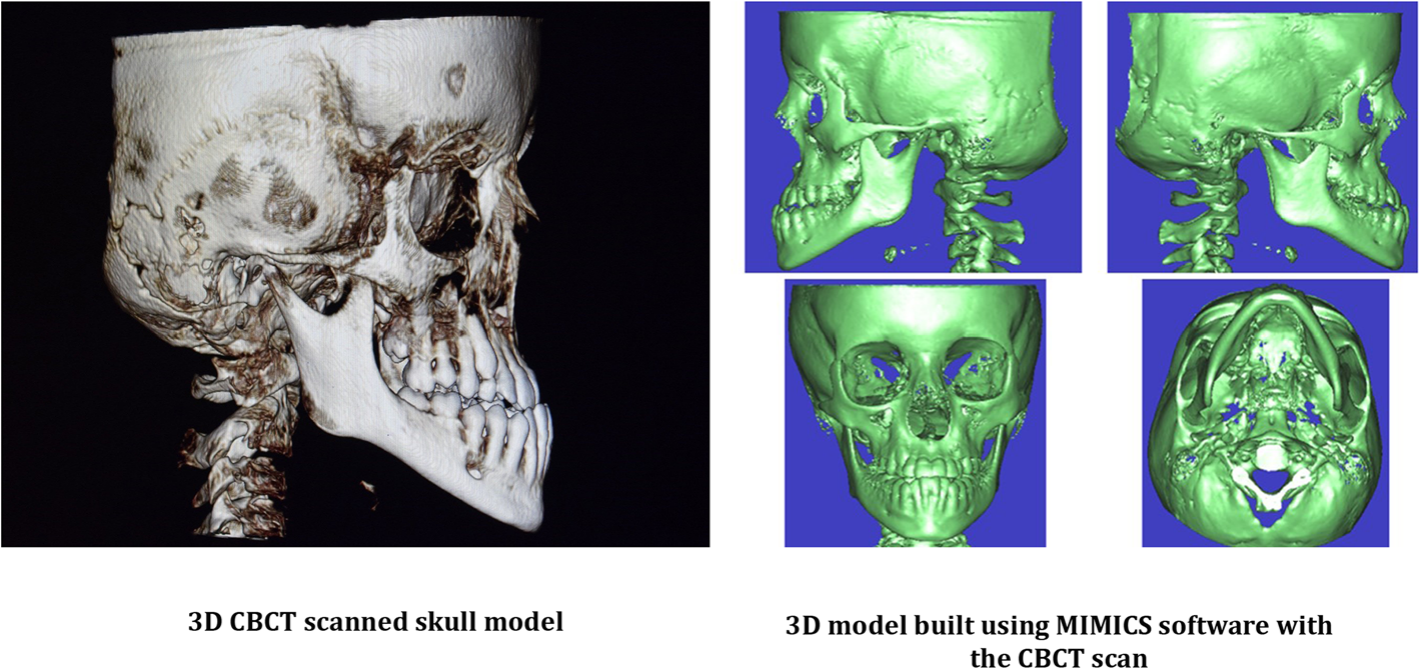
3D CBCT scanned skull model and 3D model of maxillofacial bones built by the Mimics software after the CT scan
